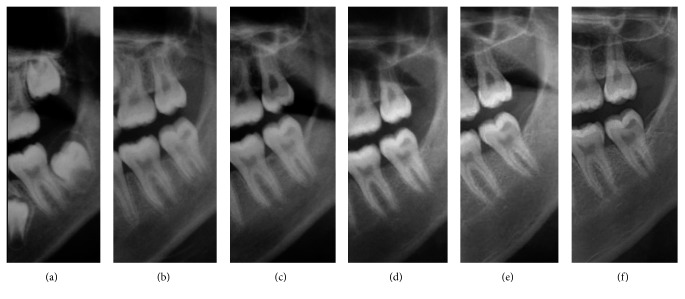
Cropped panoramic radiographs demonstrating a PEIR-affected tooth (permanent mandibular left second molar) when the patient was (a) 8 years, 8 months old, (b) 10 years, 11 months old, (c) 12 years, 5 months old, (d) 13 years, 4 months old, (e) 15 years, 3 months old, and (f) 17 years, 4 months old. There was no obvious progression of PEIR size or changes in the PEIR location noted from this film series.
